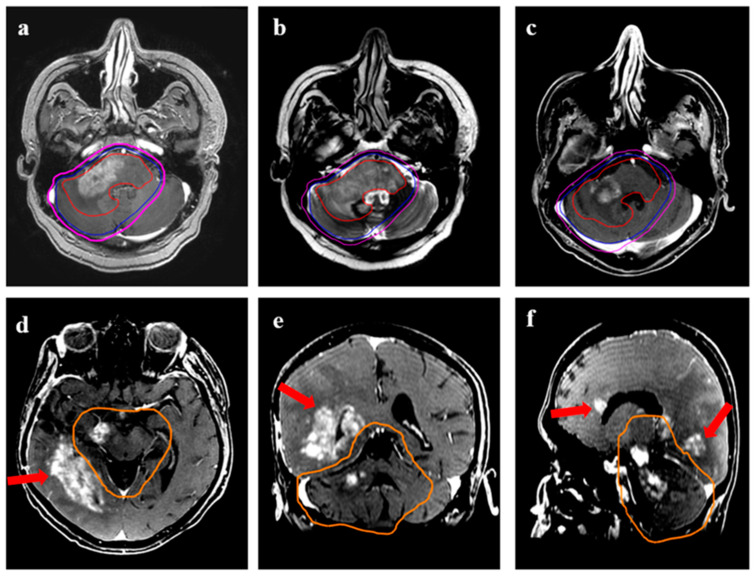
Distant relapse following re-irradiation in a 38-year male with IDH-mutant astrocytoma with transformation (radiological) to grade 4 during reirradiation. (a,b) show the target volumes (GTV: red, CTV: blue; PTV: magenta) on T1w contrast and T2w images. The GTV encompassed all the T2w altered signals, including the enhancing disease, while CTV was 10–15 mm expansion along the brain parenchyma. (c) represents the response in the primary re-irradiated region 6 months following ReRT, while the lower panel shows axial (d), coronal (e), and sagittal (f) T1w contrast MRI from the same time-point demonstrating distant areas of recurrent disease (arrow) beyond the 95% isodose line (orange).
